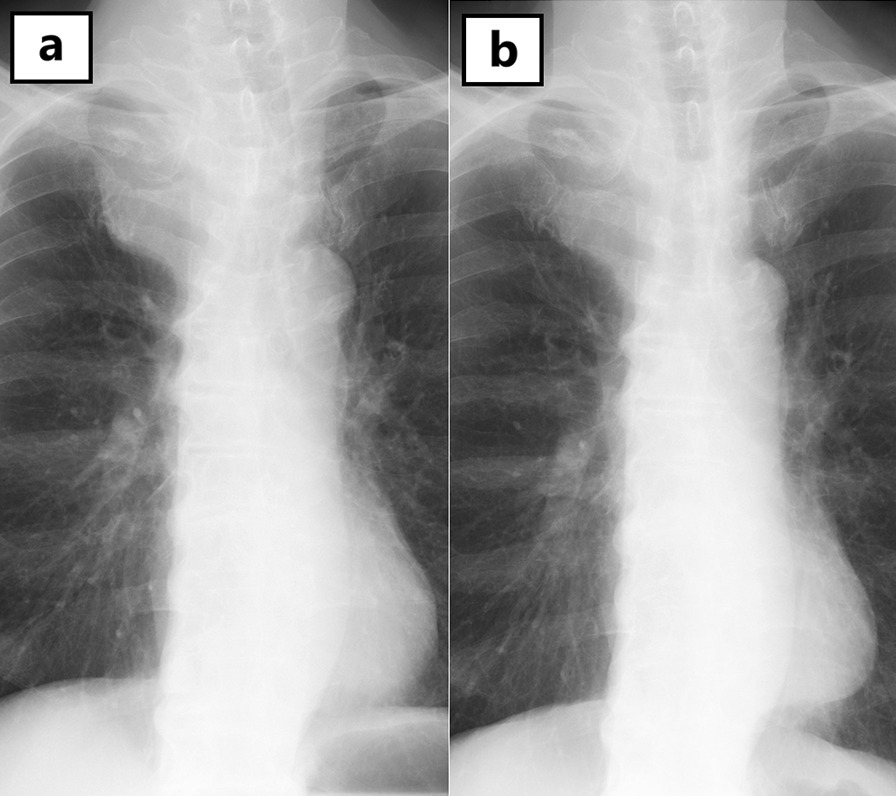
A chest radiograph showed a left tracheal deviation (a). A tracheal deviation disappeared on a chest radiograph on the third day of the first cycle (b)
